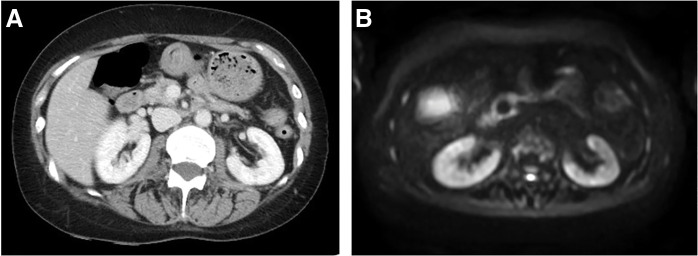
Follow-up CT shows (A) a significant reduction in the size of the pancreatic metastasis, and (B) MRI reveals that the mass in the pancreatic head has almost disappeared.
